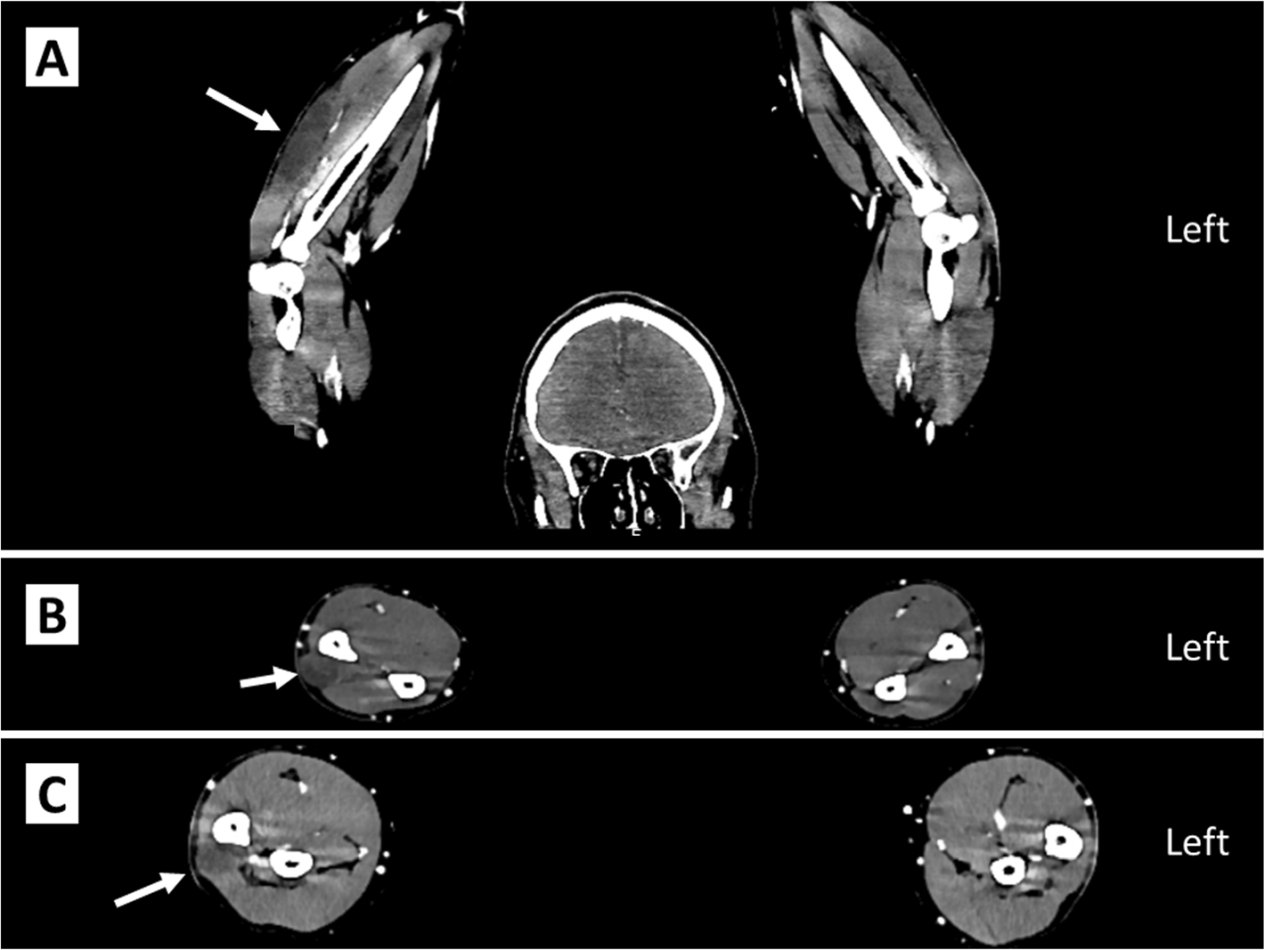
Preoperative computed tomography scan with *arrows* pointing at dark areas in the right extensor carpi ulnaris muscle, indicating edema. a Coronal plane; b transverse plane, middle; c transverse plane, proximal
